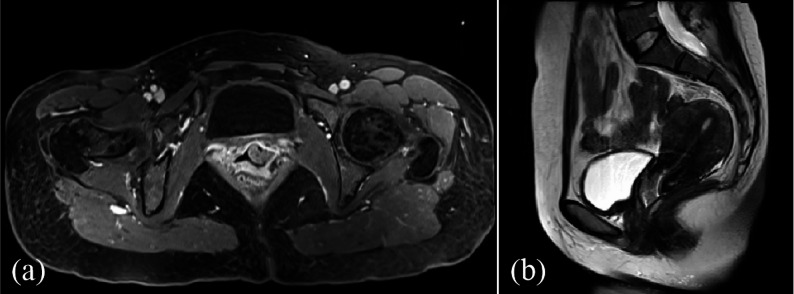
Representative MRI images: (a) axial fat-suppressed T1-weighted imaging and (b) sagittal T2-weighted imaging
